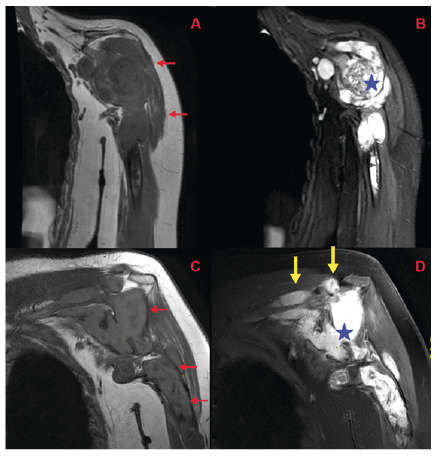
Coronal T1-weighted and PD images at diagnosis (A and B) and corresponding coronal oblique T1-weighted and PD images after 10 years of follow-up (C and D) demonstrate progression of bone-based lesions despite routine treatment (red arrows). Soft tissue involvement also progresses, affecting the joint at the humeral head (blue asterisks). In the current imaging, lesions are more clearly identifiable within the supraspinatus and deltoid muscle planes (yellow arrows).
